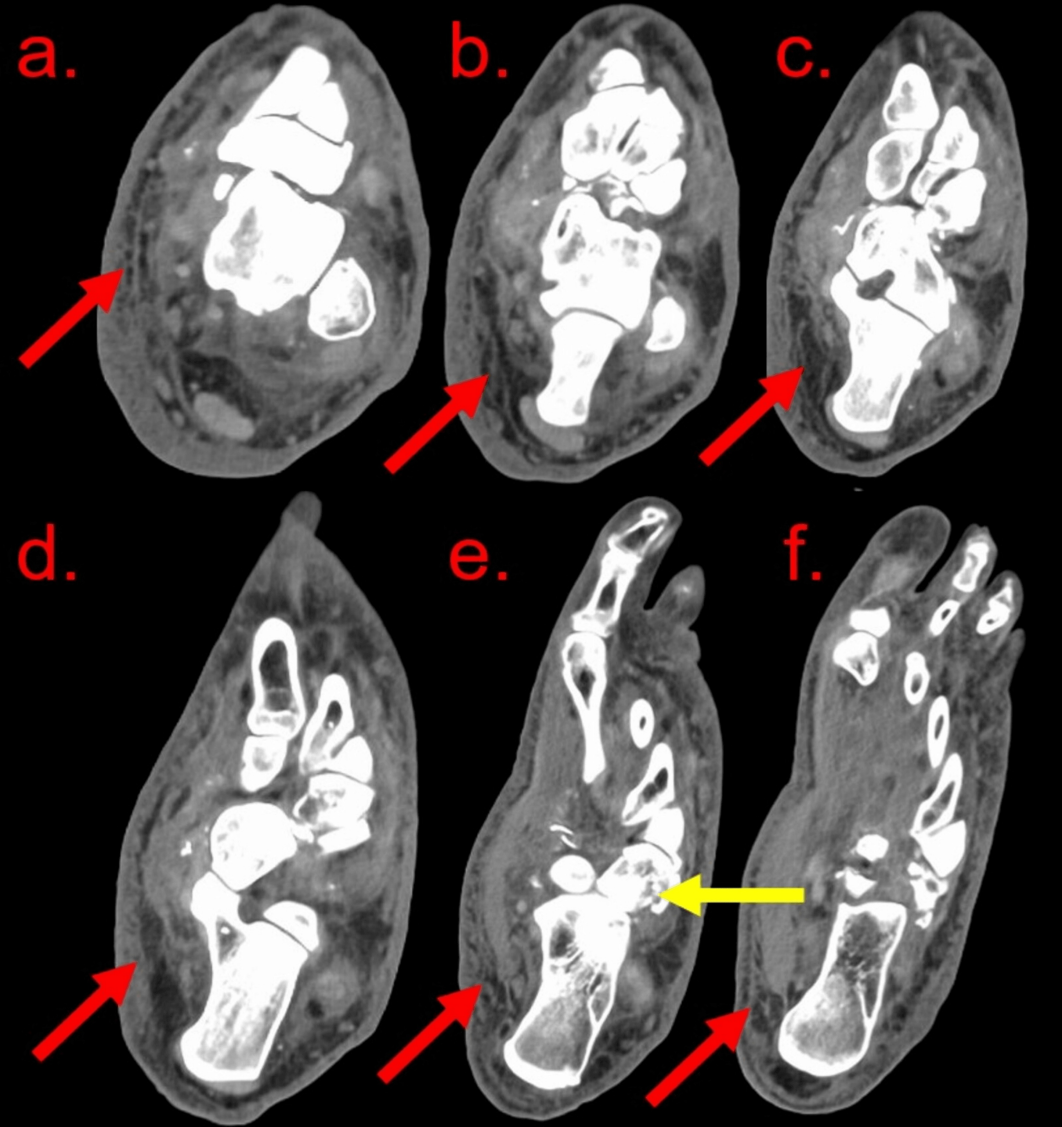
A CT scan without contrast of the left foot and ankle A CT scan without contrast of the left foot and ankle reveals diffuse subcutaneous fat stranding (red arrows) and edema throughout the ankle and foot. There is dorsal dislocation of the talonavicular joint and multiple small bony fragments along the superior and inferior margins of the navicular bone. There are markedly comminuted acute intra-articular fractures of the cuboid (yellow arrow) with disruption of the calcaneocuboid joint.
